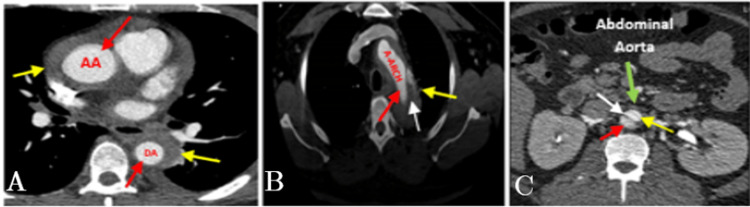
CT of the aorta demonstrates Stanford type A aortic dissection. (A) Ascending aorta. (B) A-arch: the red arrow showing the true lumen, the yellow arrow showing the false lumen, and the white arrow showing the flap. (C) The blue arrow showing the abdominal aorta, the red arrow showing the true lumen, and the yellow arrow showing the false lumen. CTA = computed tomography; DA = descending aorta; AA = ascending aorta; A-arch = aortic arch
